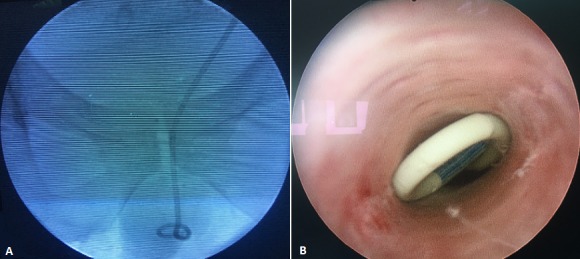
A) X-ray showing the lower loop of the double j stent out of the bladder; B) uretroscopy showing the lower loop of the double j stent at the anterior ureter
